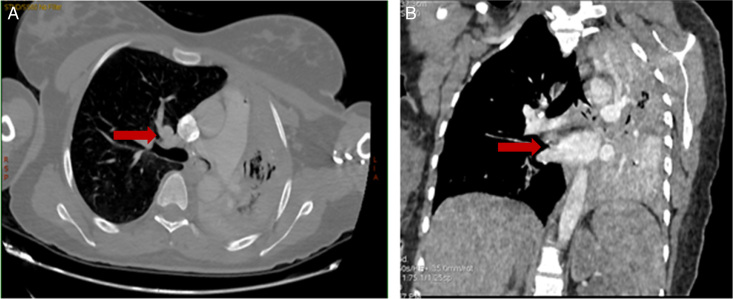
(A) Computed tomography (CT) chest: axial view showing left main stem bronchus obstruction (red arrow) with left sided atelectatic changes. (B) CT chest: coronal view showing left main stem bronchus occlusion (red arrow).
